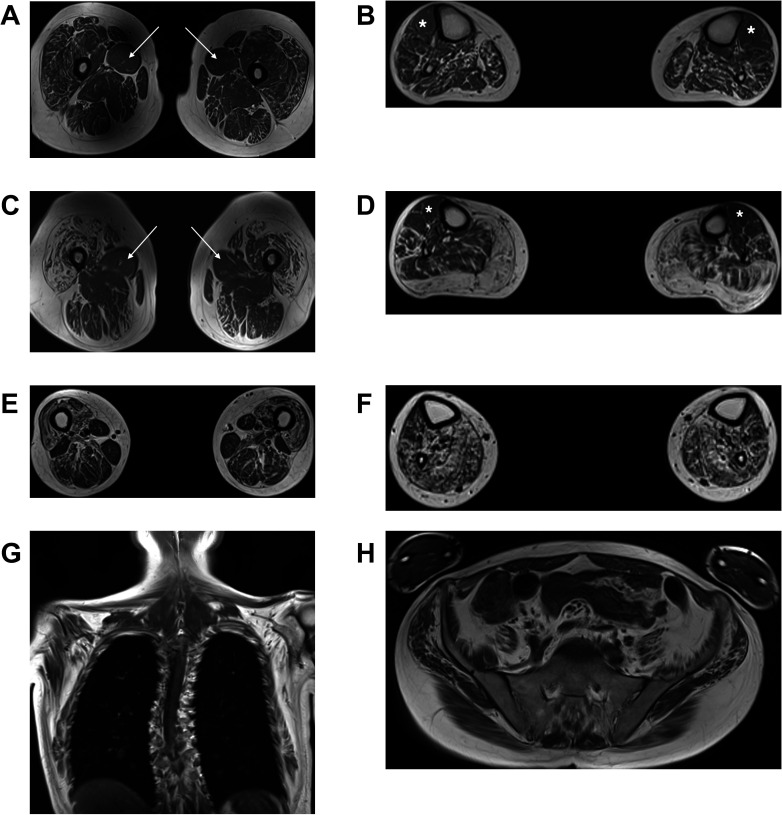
Muscle MR images in patients with Charcot-Marie-Tooth disease type 2CC. The panels show T1-weighted MR images of the thighs and calves in individuals UK1-IV.1 (A, B), UK4-IV.7 (C, D) and FR2-II.1 (E–H). In all three patients, the degree of intramuscular fat accumulation in the thighs is similar to that in the calves. In individual UK1-IV.1 at the thigh level (A), there is streaky fatty change particularly involving vastus lateralis, vastus intermedius and rectus femoris, with conspicuous sparing and relative hypertrophy of adductor longus (white arrows). In the calves (B), there is generalised intramuscular fatty change, more pronounced in the posterior compartment, with relative sparing of the tibialis anterior (white asterisks). In individual UK4-IV.7 at the thigh level (C), there is severe fatty degeneration of the quadriceps with small ‘islands’ of preserved motor units indicating advanced neurogenic amyotrophy. Again, there is relative sparing of the adductor longus (white arrows). In the calves (D), there is almost complete fatty replacement of the gastrocnemius, with less marked streaky fatty changes elsewhere and relative sparing of the tibialis anterior (white asterisks) and the extensor digitorum longus. In individual FR2-II.1, who clinically and radiologically is the most severely affected of the three patients, the thigh images (E) show severe fatty change of the quadriceps, with less marked changes involving the posterior compartment. In the calves (F), there is severe fatty change affecting the posterior compartment muscles, with slightly less severe involvement of the tibialis anterior and the extensor digitorum longus. Whole body T1-weighted MR images acquired in this individual (G, H) also demonstrate widespread symmetric involvement of the shoulder girdle, chest wall and pelvic muscles. MR, magnetic resonance.
